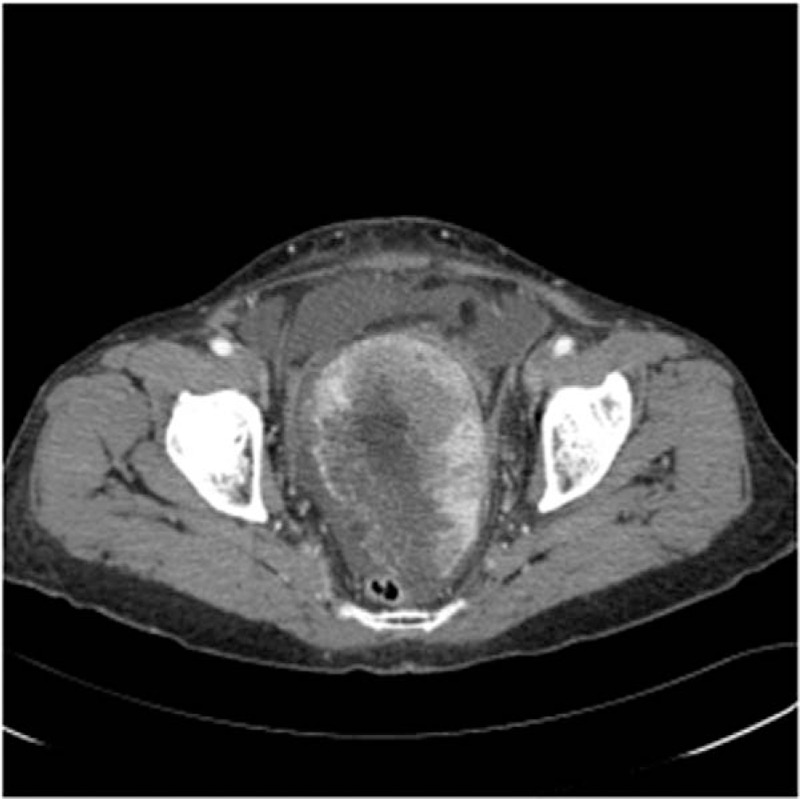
Abdominal CT scans for the patient. CT scan showed massive ascites with a pelvic mass measuring 12 × 7.8 × 8.2 cm. The pelvic mass consisted of multi-lobulated, cystic, and solid contents.
